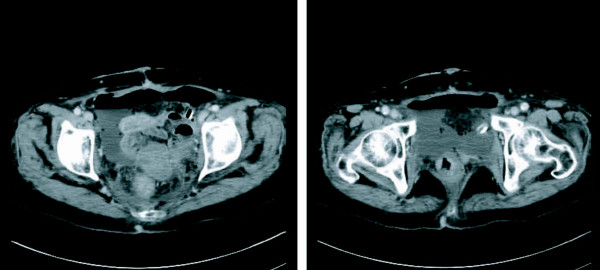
Computed tomography images on postoperative day three. Fluid collection and free air is visible at the previous surgical resection site.
